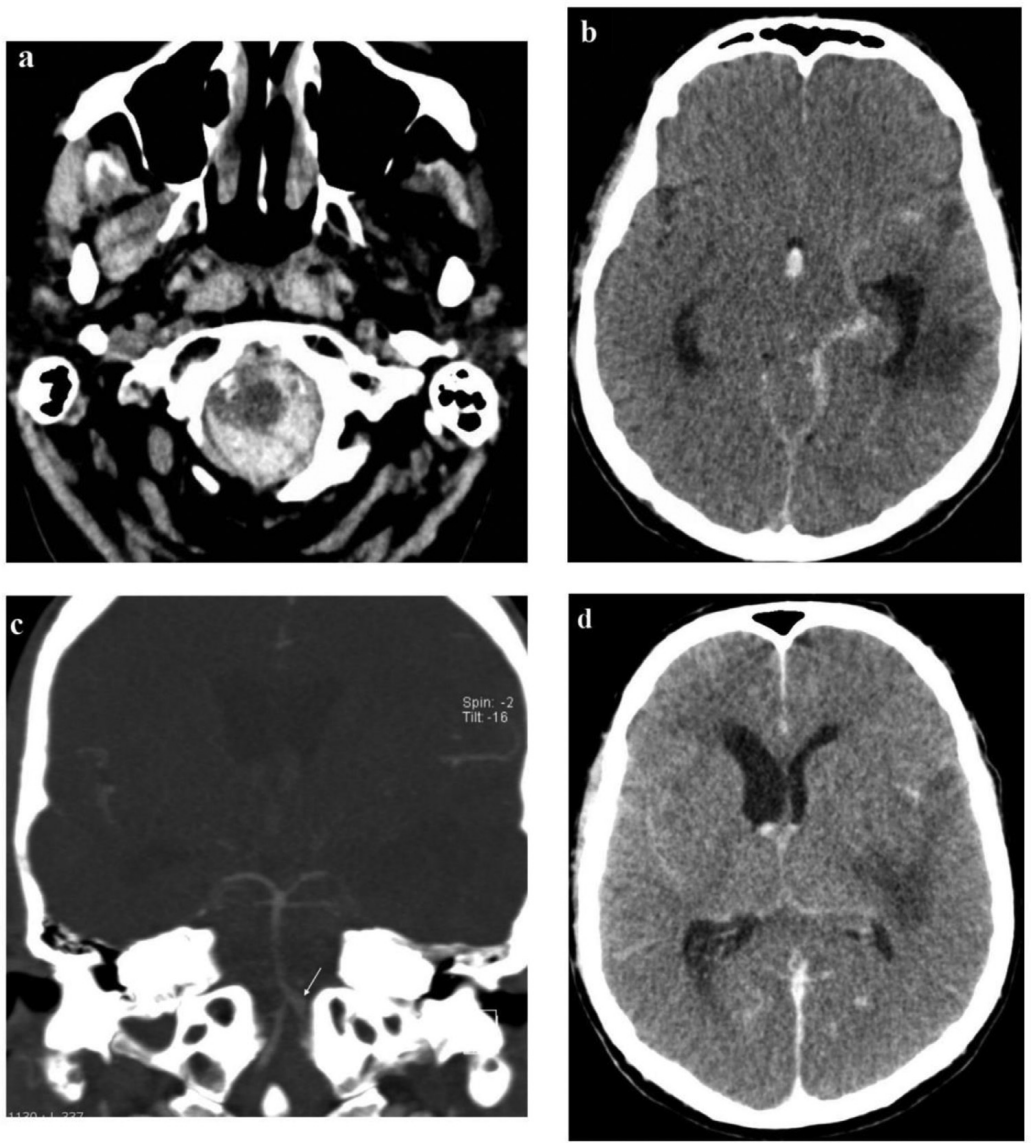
(Hospital day 16). (A) Head CT in axial plane shows subarachnoid hemorrhage in foramen magnum. (B) Subarachnoid hemorrhage in the prepontine and interpeduncular cisterns, left ambient cistern, and left sylvian fissure. (C) CTA of intracranial arteries reveals a fusiform dilatation of the left vertebral artery (arrow). (D) Repeated head CT 12 hours after resuscitation shows progression of subarachnoid hemorrhage with blood in the cerebral sulci and in the third and fourth cerebral ventricle, along with development of diffuse cerebral edema.
